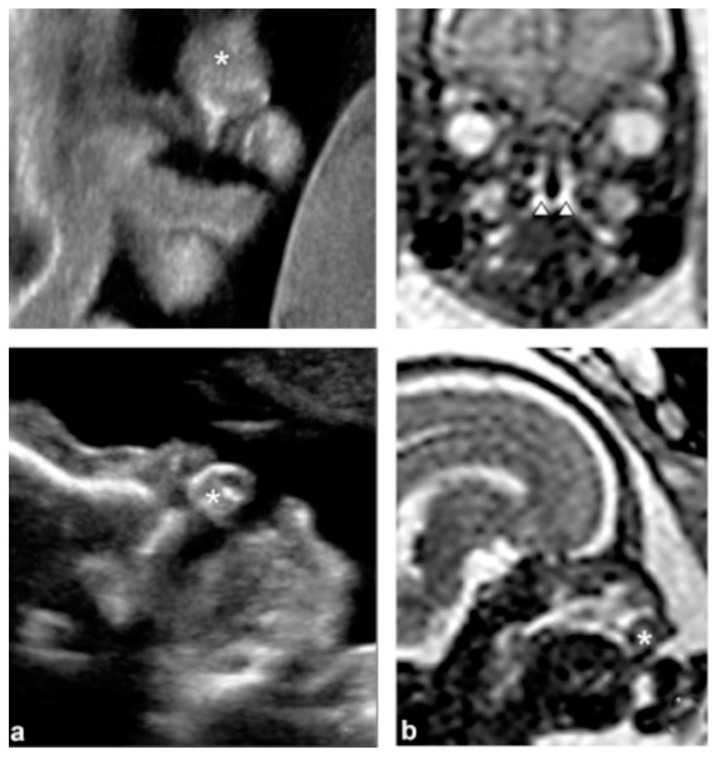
Bilateral cleft lip and alveolus and palate. (a) Coronal and sagittal views using 2D ultrasound. * premaxillary protrusion (b) Magnetic resonance imaging (MRI) images in the coronal and sagittal planes. * premaxillary protrusion; arrowheads: cleft palate. Reprinted with permission from Ref. [3]. Copyright ® 2016 Elsevier Masson SAS All rights reserved.
